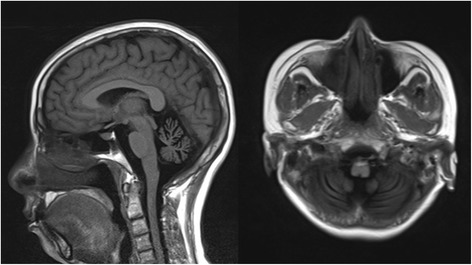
Brain MRI images for the proband (IV2). Marked and diffuse cerebellar atrophy was present in the proband
